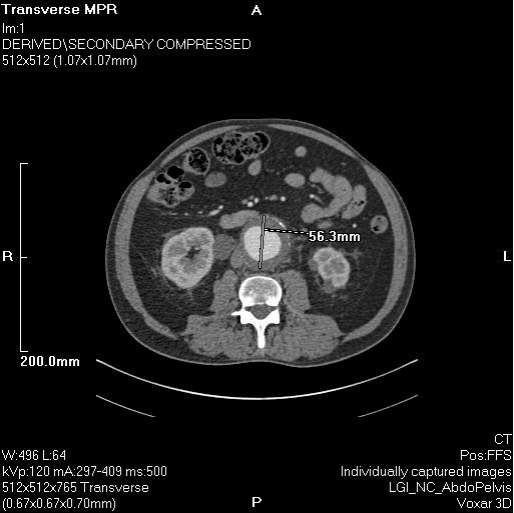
CT aortogram demonstrating a 5.6 cm anteroposterior diameter AAA.
